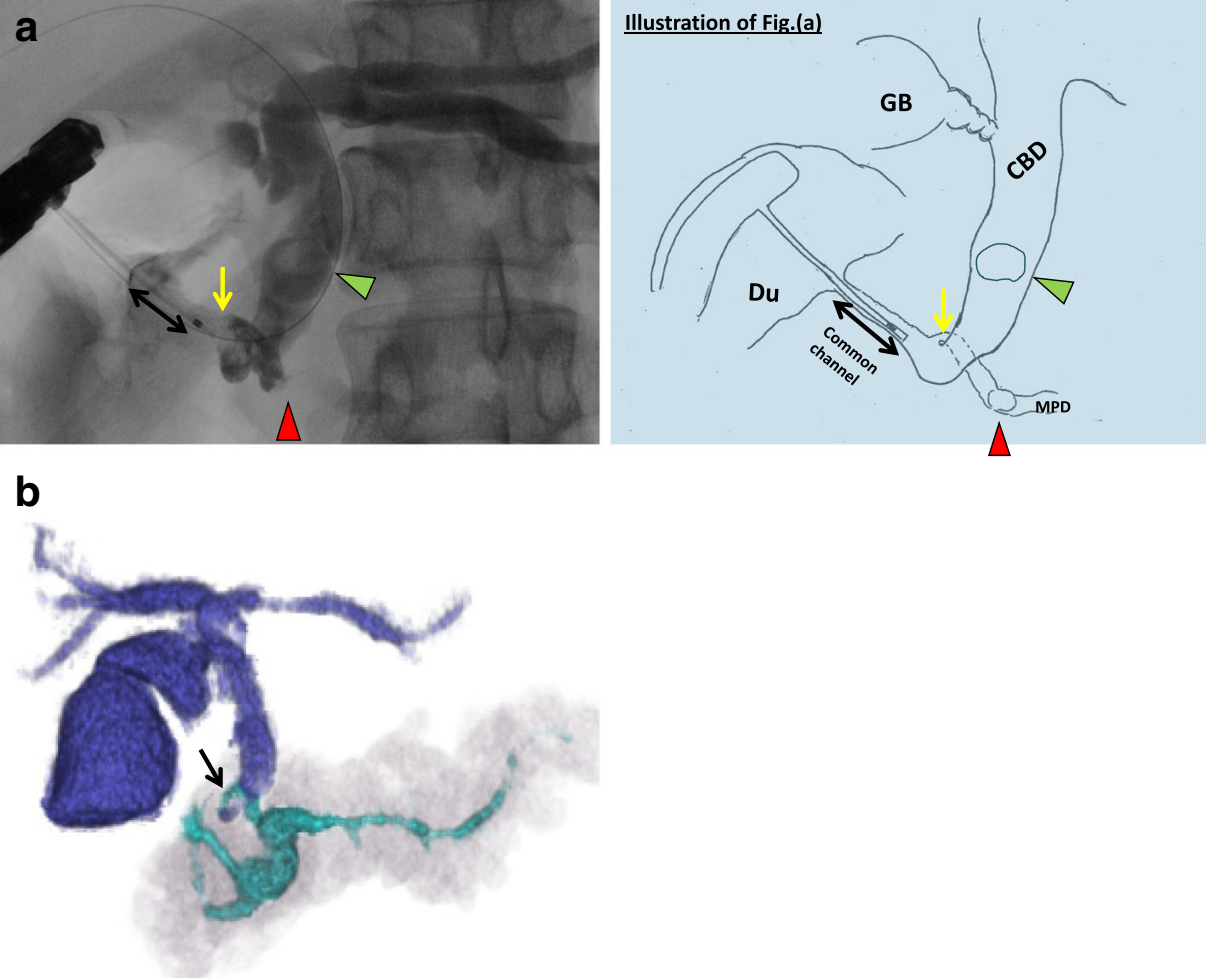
Findings of endoscopic retrograde cholangiopancreatography and three-dimensional cholangiopancreatography by computed tomography data. A filling defect in the common bile duct due to choledocholithiasis (green triangle) and the main pancreatic duct in the pancreas head (red triangle), detected by endoscopic retrograde cholangiopancreatography; ERCP (a). And, the pancreatic duct joined near the lower common bile duct (arrow), and the common channel (two-way arrow) was revealed. Three-dimensional cholangiopancreatography (b) revealed joint portion of pancreatic duct and common bile duct and annular pancreatic duct. Pancreas duct distal to the filling defect was slightly dilated
